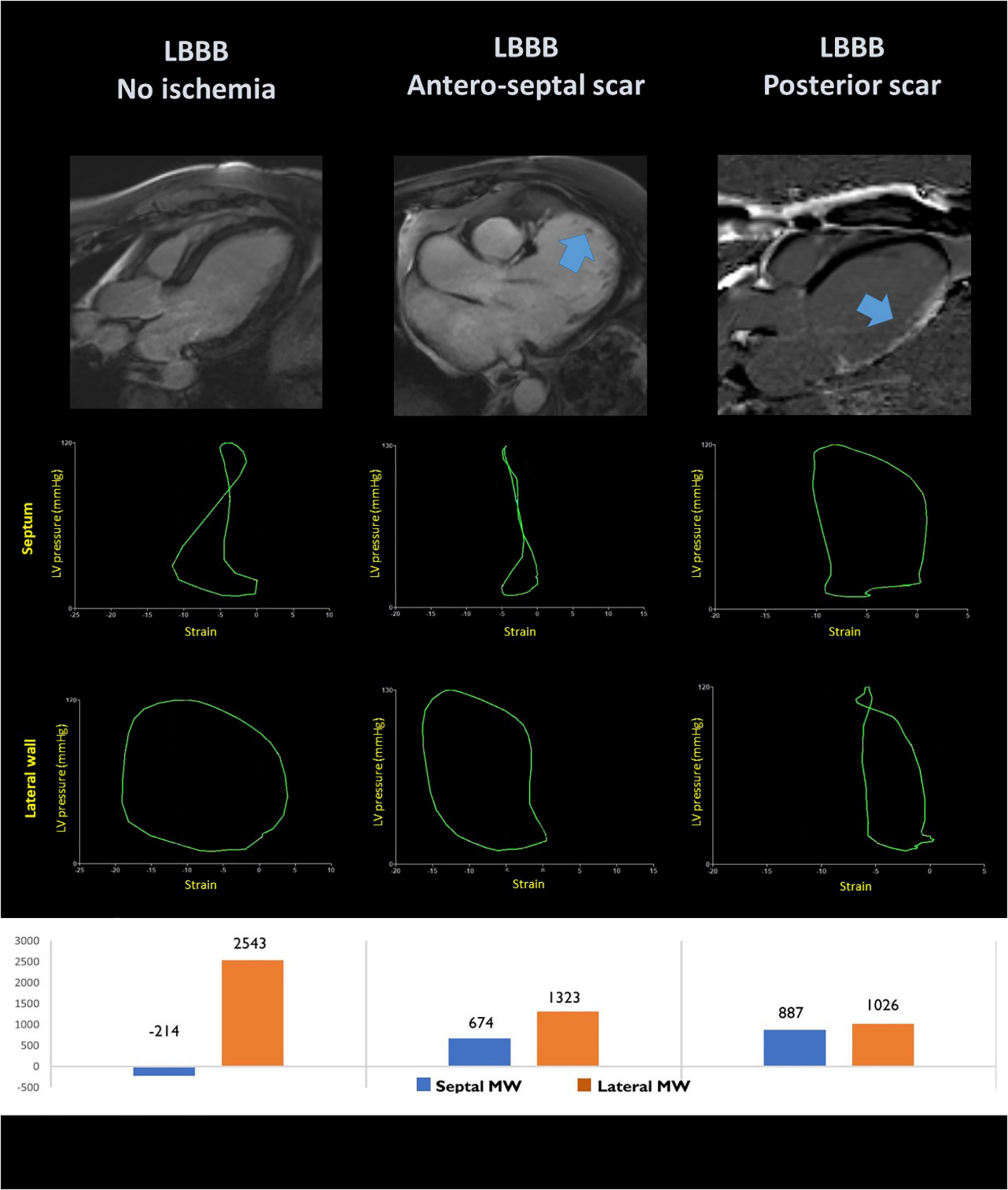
Pressure-loops analysis in the septal and lateral segments of patients with left bundle branch block without ischemic heart disease (left panel); with anteroseptal scar (central panel) and lateral wall scar (right panel). The corresponding localisation of the myocardial scar is shown at MRI late gadolinium enhancement in the upper panels.
